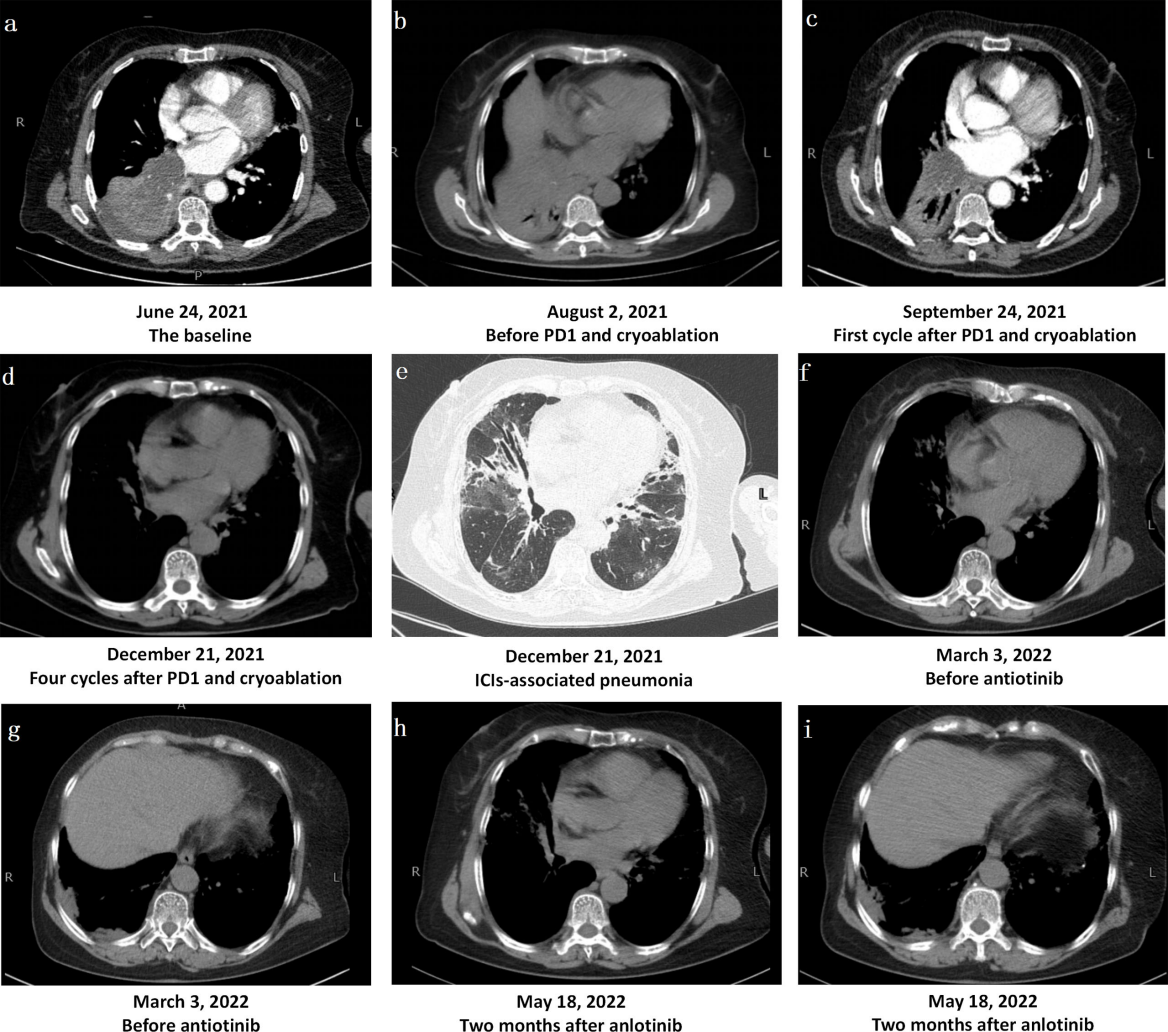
Changes in Computerized Tomography Images. (A) The bronchus of the lower lobe of the right lung was truncated from hilus of lung, and the basal segment of the lower lobe of the right lung was clumped with consolidation shadow and obstructive atelectasis. (B) Obstructive pneumonia and atelectasis were worse after one-cycle treatment of bevacizumab combined with albumin-bound paclitaxel and carboplatin. (C, D) The mass in the lower lobe of the right lung was significantly reduced, obstructive pneumonia and atelectasis were significantly improved after PD1 and chemotherapy. (E)After treatment, there were multiple consolidation shadows, ground glass shadows and interstitial changes in chest CT, which was considered as immune checkpoint inhibitor-associated pneumonia. (F, G). After three months of methylprednisolone administration, the tumor near the pleura in the lower lobe of the right lung progressed slightly, and the immune pneumonia was not completely resolved. (H, I) After anlotinib treatment, the tumor near the pleura in the lower lobe of the right lung was stable.
